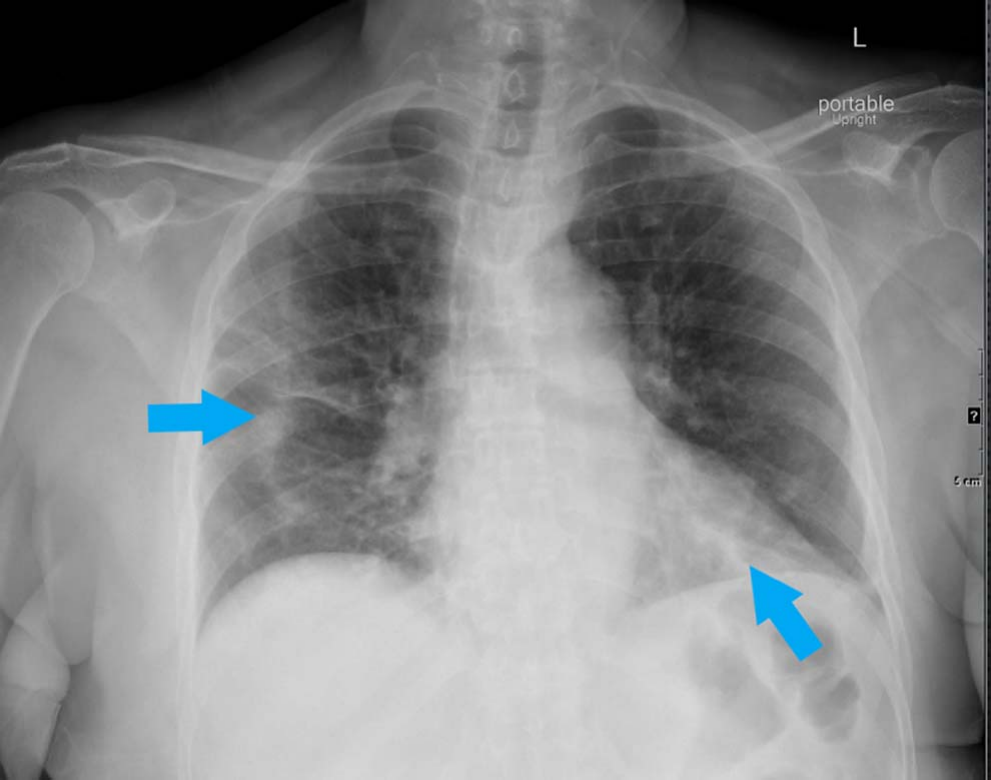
Portable upright anteroposterior radiograph of a 72-year-old female shows characteristic bilateral confluent, bandlike (arrows) consolidative opacity in periphery of the right mid- and left-lower lung zones.
